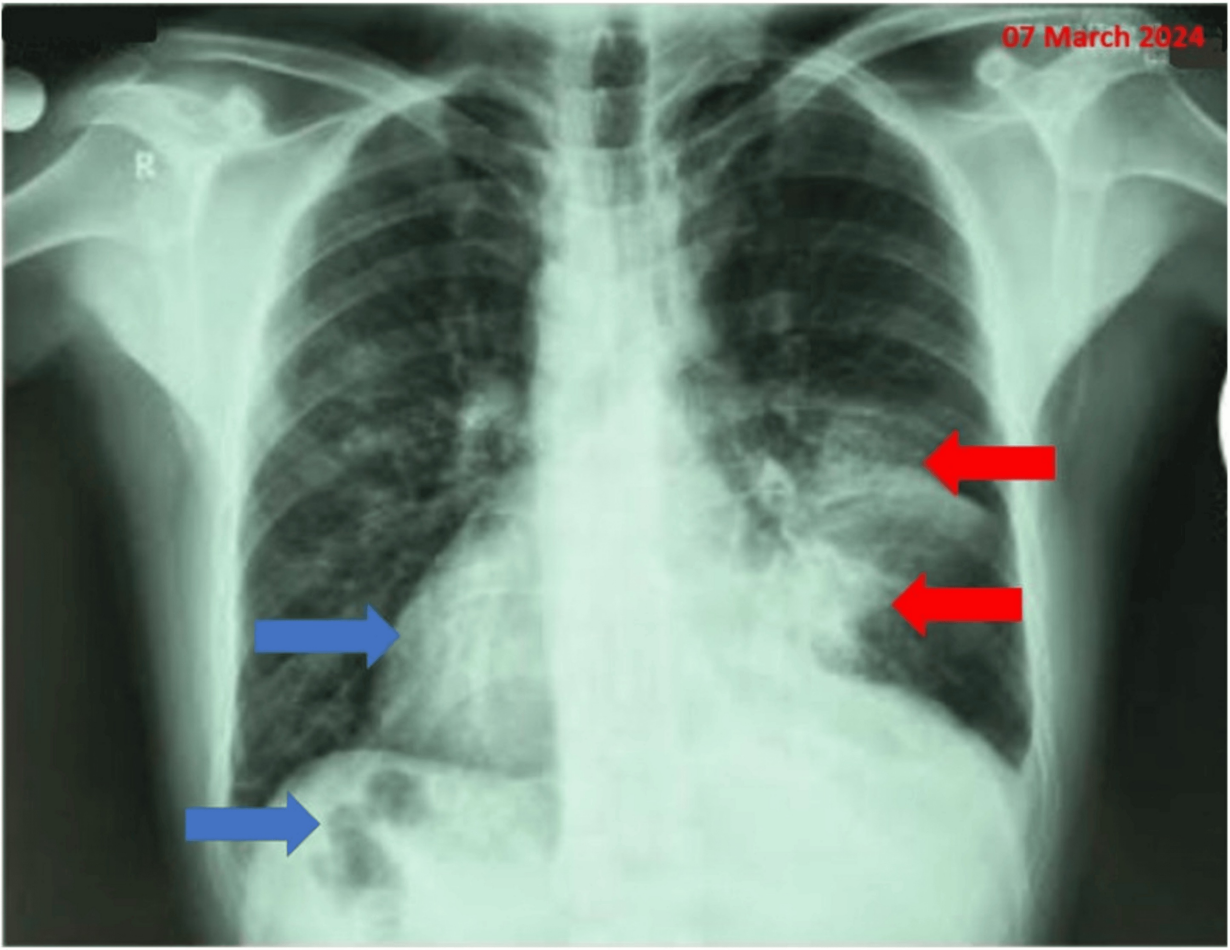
Chest X-ray revealing malposition (mirrored anatomy) characteristic of SIT
